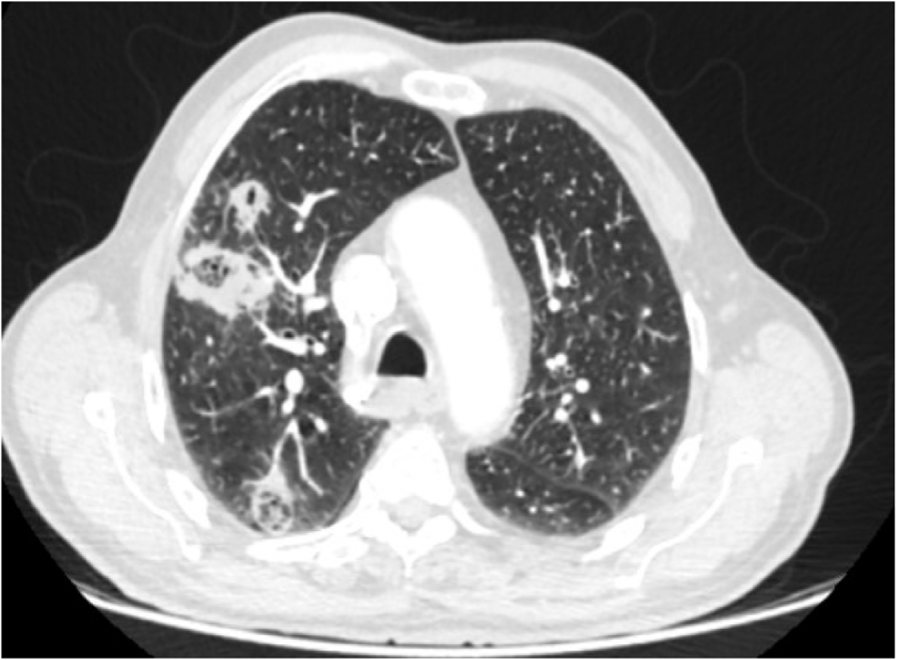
Pulmonary zygomycosis: thin section CT shows multiple nodules with reversed halo sign (RHS) in the right upper and lower lobes
